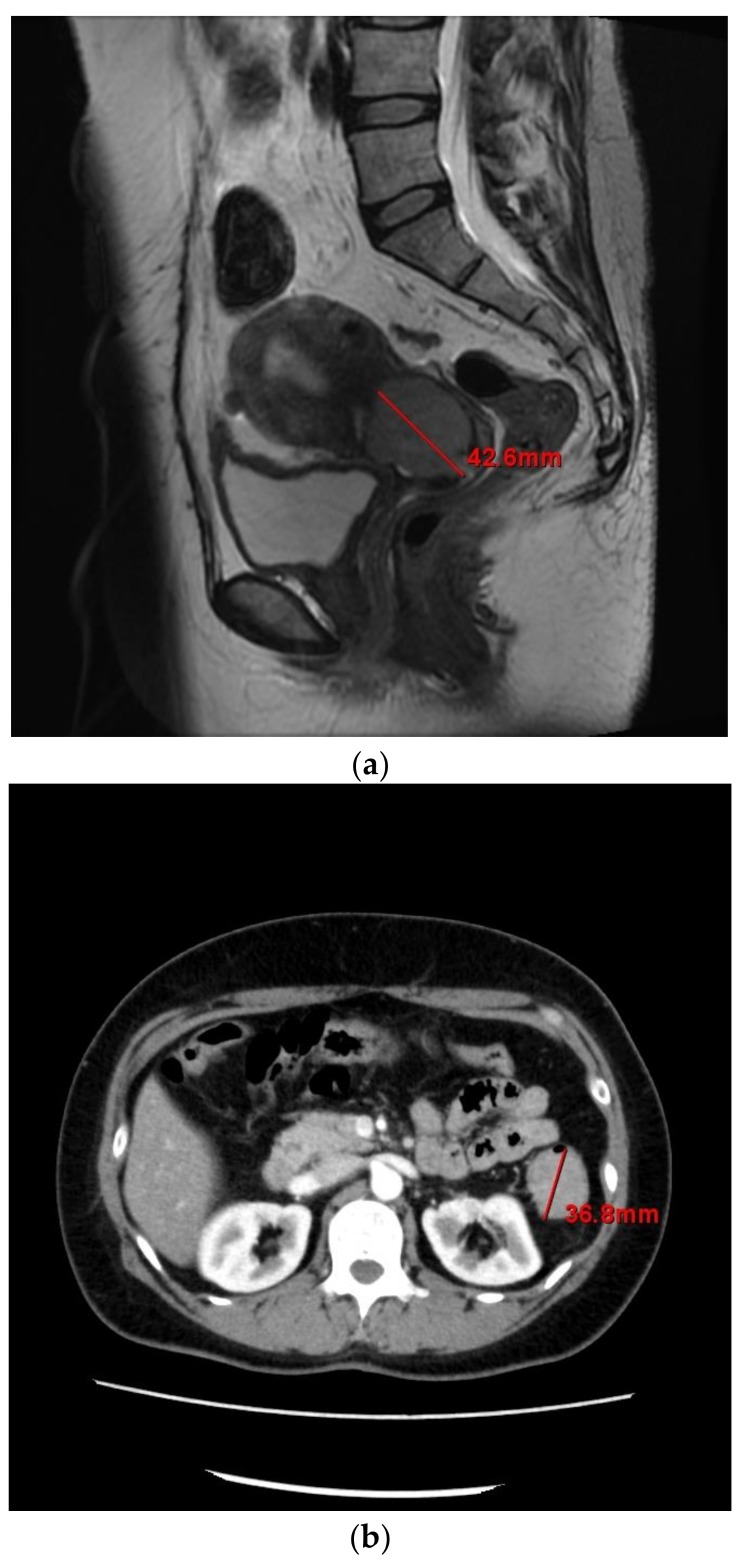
(a) Pelvic magnetic resonance imaging of the tumor in the endocervix; (b) Contrast-enhanced computed tomography of the abdomen showing the transverse colon tumor.
